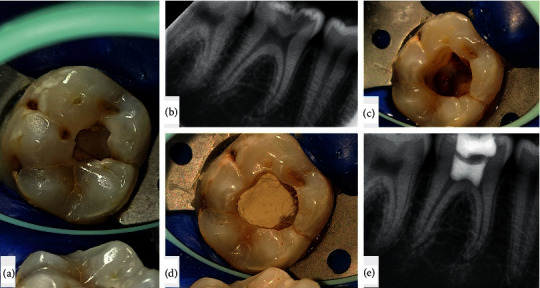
Stepwise procedure of pulpotomy. Step a, preoperative picture of the affected tooth. Step b, preoperative radiograph of the tooth. Step c, removal of the affected coronal pulp and leaving the radicular pulp intact. Step d, placement of MTA. Step e, postoperative radiograph.
